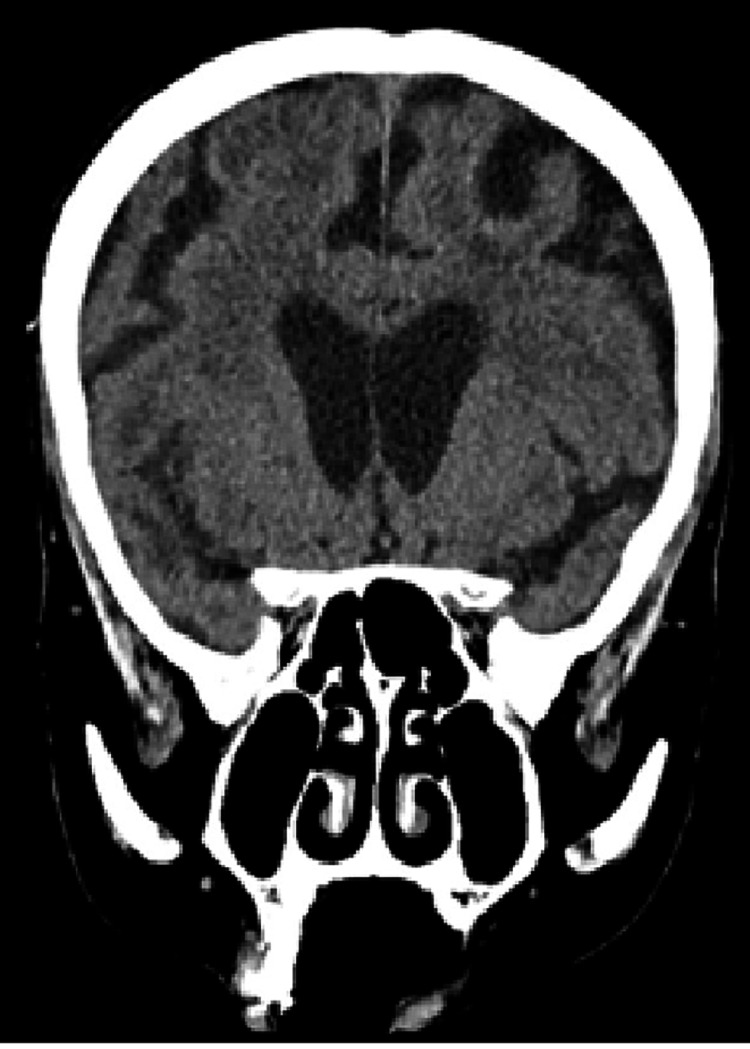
Coronal CT brain on day one of admission demonstrating moderate periventricular hypodensity in keeping with chronic small vessel ischemia and age-appropriate involutional changes aside from prominence of sulci within the left hemisphere, suggestive of prior infarction.
